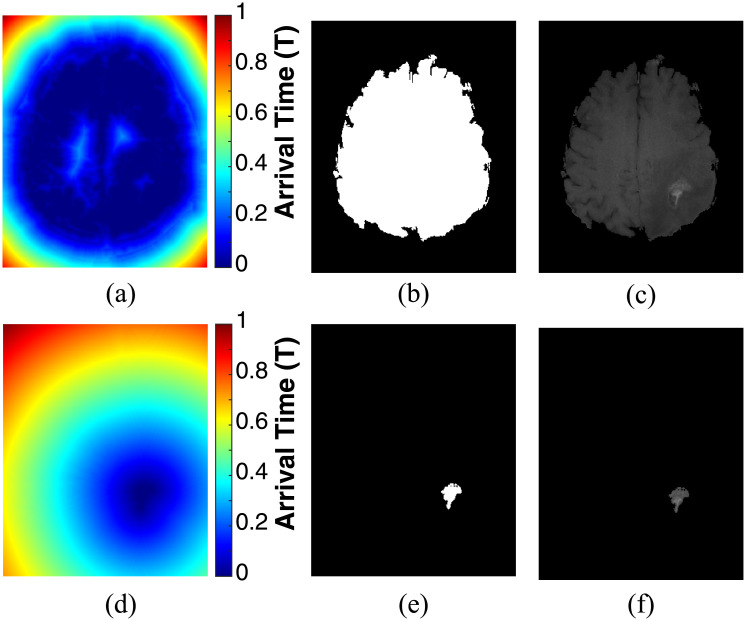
Application of FMM on the MRI slice shown in Fig 5(a): (a) arrival time estimation for the brain tissue, (b) segmented binary brain tissue, (c) segmented gray-level intensity brain tissue, (d) arrival time estimation for the tumor area, (e) segmented binary tumor area, and (f) segmented gray-level intensity tumor area.
